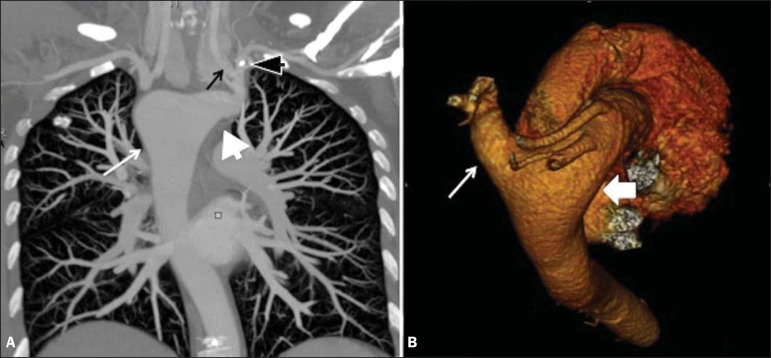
A: Chest computed tomography angiography, maximum intensity
